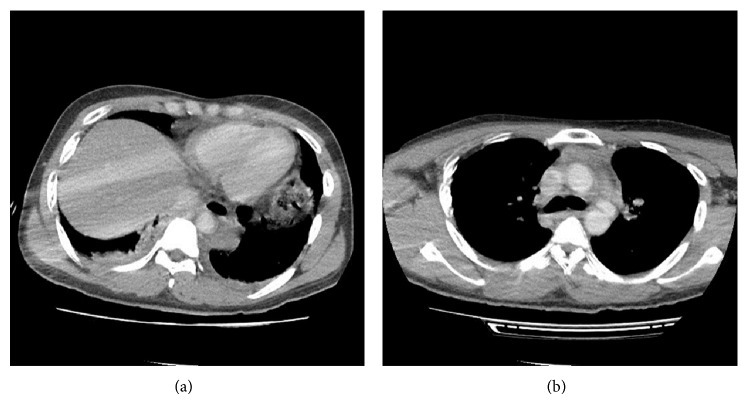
Computed tomogram showing (a) 2 × 1 cm contrast-filled outpouching at the anterolateral aspect of the distal aortic arch with (b) associated mediastinal fat stranding and crescentic soft tissue density along the descending thoracic aorta.
